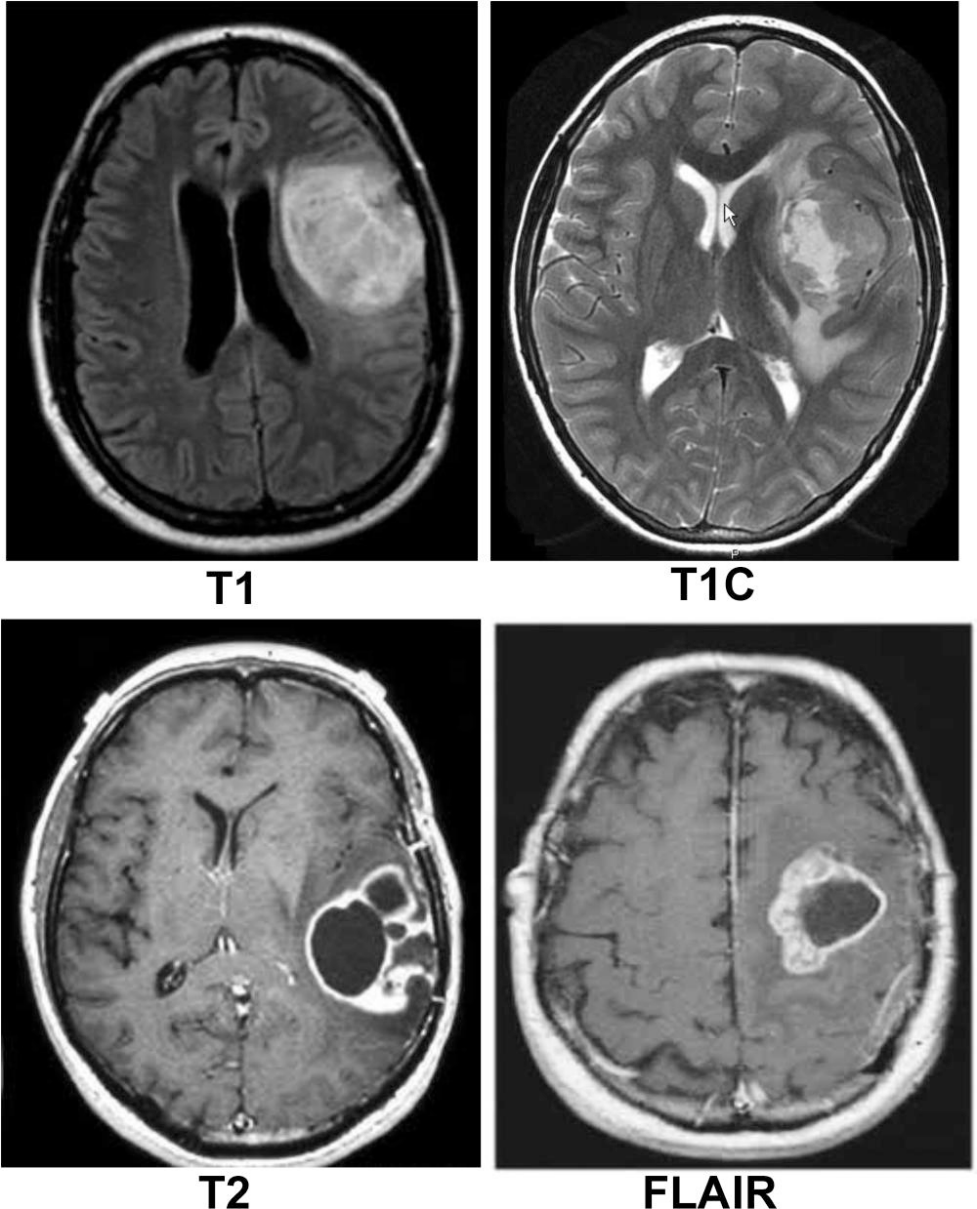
Various MRI slices of the brain. T1-weighted image (T1): Provides clear visualisation of the anatomy, showing high contrast between different tissues, particularly between grey and white matter. Used for identifying anatomical abnormalities of the brain; T1-weighted contrast-enhanced image (T1c): Similar to T1 images but with the addition of a contrast agent, usually gadolinium, which enhances the visibility of blood–brain barrier disruptions, tumours and other pathologies; T2-weighted image (T2): Highlights differences in the water content of tissues, making it useful for identifying oedema, inflammation and other conditions associated with increased water content, such as tumours or cysts. T2 images show fluid (like cerebrospinal fluid) as bright and white matter as dark; FLAIR: A special type of T2-weighted image where the signal from cerebrospinal fluid is suppressed, making it easier to see lesions near the ventricles and cortex, such as in cases of multiple sclerosis or brain tumours).
